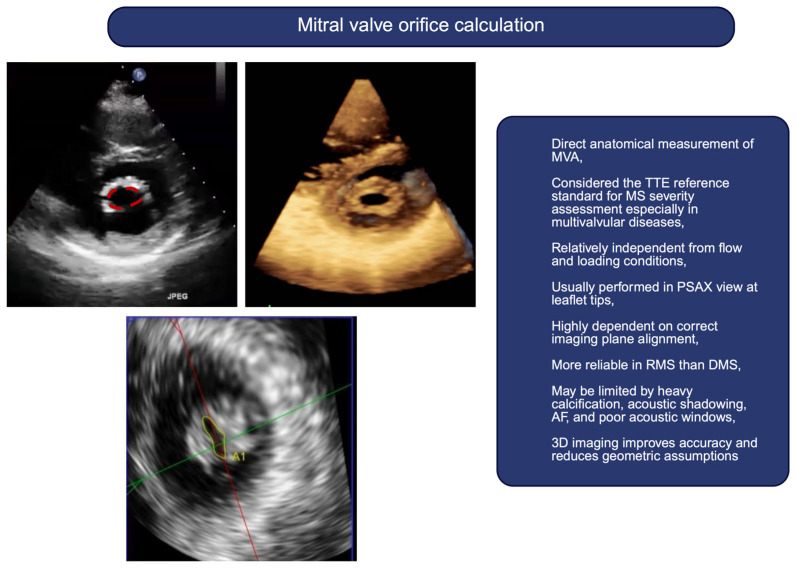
2D and 3D TTE planimetry of the MVA in PSAX view at the level of the leaflet tips. Direct tracing of the mitral orifice (red dashed contour) allows anatomical quantification of MVA and represents the TTE reference standard for MS severity assessment. Although relatively independent from flow conditions, planimetry accuracy is highly dependent on optimal imaging plane alignment and may be limited by calcification, acoustic shadowing, atrial fibrillation, and irregular valve geometry. Abbreviations: AF: atrial fibrillation, DMS: degenerative mitral stenosis, MVA: mitral valve area, PSAX: parasternal short axis view, RMS: rheumatic mitral stenosis, TTE: trans-thoracic echocardiography.
